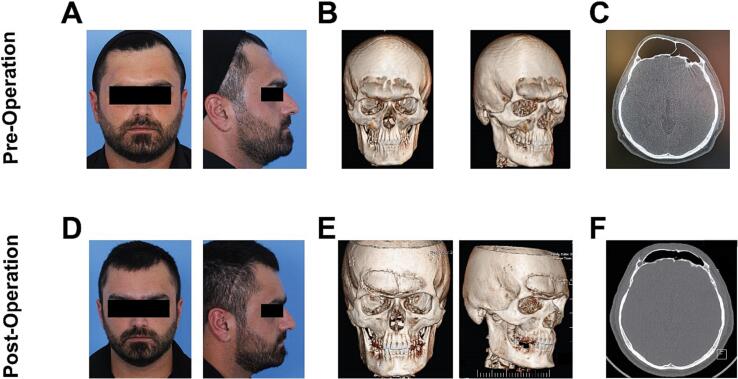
(A) Preoperative frontal and lateral views showing asymmetrical frontal bossing more sever in right side. (B) Preoperative computed tomography scan (3D frontal and right view), showing extensive pneumatization and expansion of the frontal sinuses. (C) Preoperative computed tomography scan of paranasal sinuses (lower axial view), showing expansion of the frontal sinus where the anterior table of the frontal sinus was pushed forward without any bony erosion. (D) Postoperative frontal and lateral views showing resolved frontal bossing and successful facial contouring. (E) Postoperative computed tomography scan (3D frontal and right view), showing proper bone reposition and frontal bossing correction. (F) Postoperative computed tomography scan of paranasal sinuses (lower axial view), showing normal frontal sinus structure without mucosal swelling.
